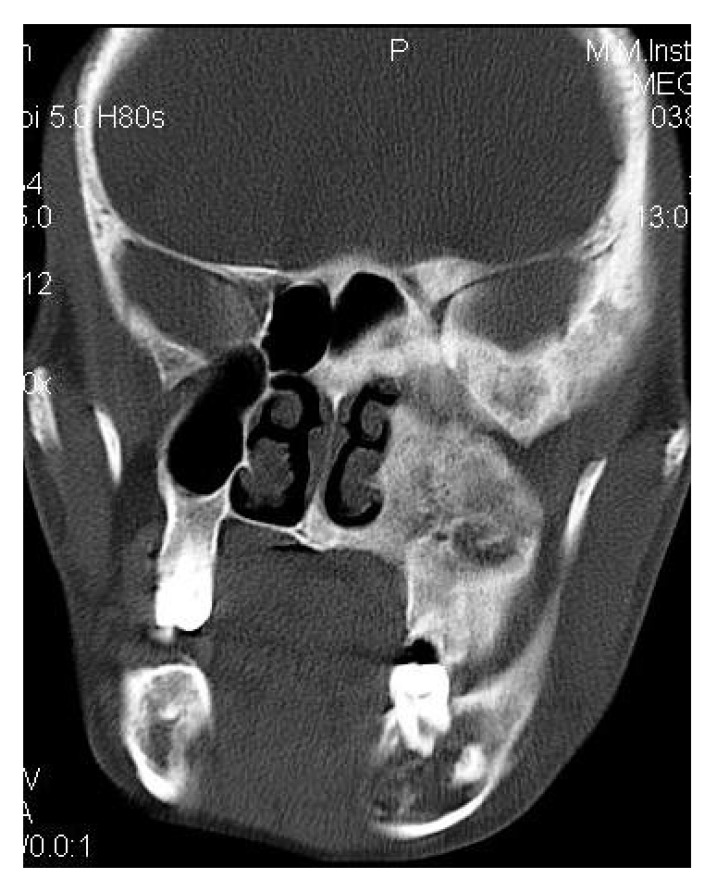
Coronal CT section showing the involvement of right side of mandible with the TMJ, glenoid fossa, temporal bone, frontal bone and maxilla.
